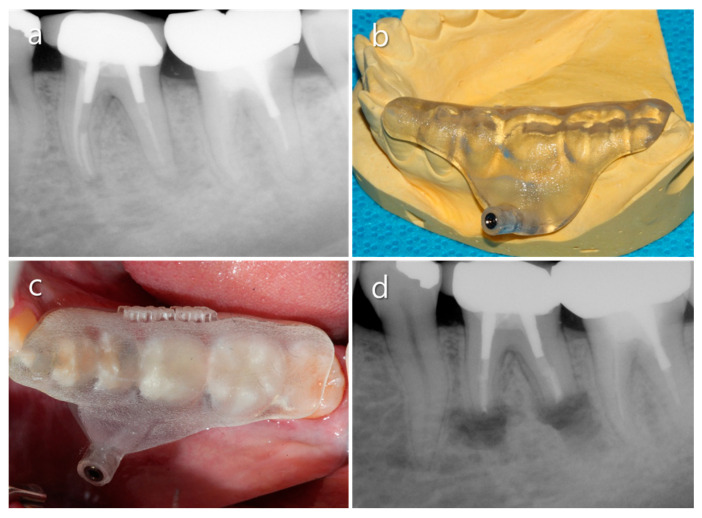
Clinical case using a computer-aided design/computer-aided manufacturing (CAD/CAM)-guided surgical template to localize the root apex through thick. (a) Diagnostic periapical X-ray. (b) A manufactured surgical template. (c) The surgical template was positioned on the tooth. (d) Postoperative radiograph.
